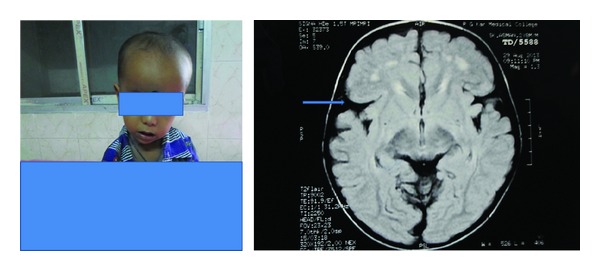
Showing patient with macrocephaly, typical facies, and MRI of his brain reveals frontotemporal atrophy, dilated sylvian fissures with open opercula (arrow), diffuse hyperintense lesions in bilateral basal ganglia, and both frontal white matter and bilateral periventricular area. Widening of the sylvian fissure gives the characteristic “bat-wing” appearance.
